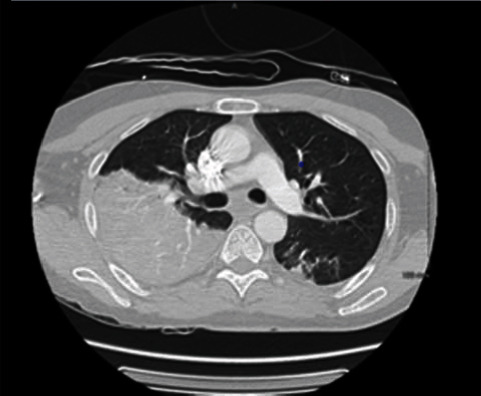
CT scan: right lower lobe consolidation.
